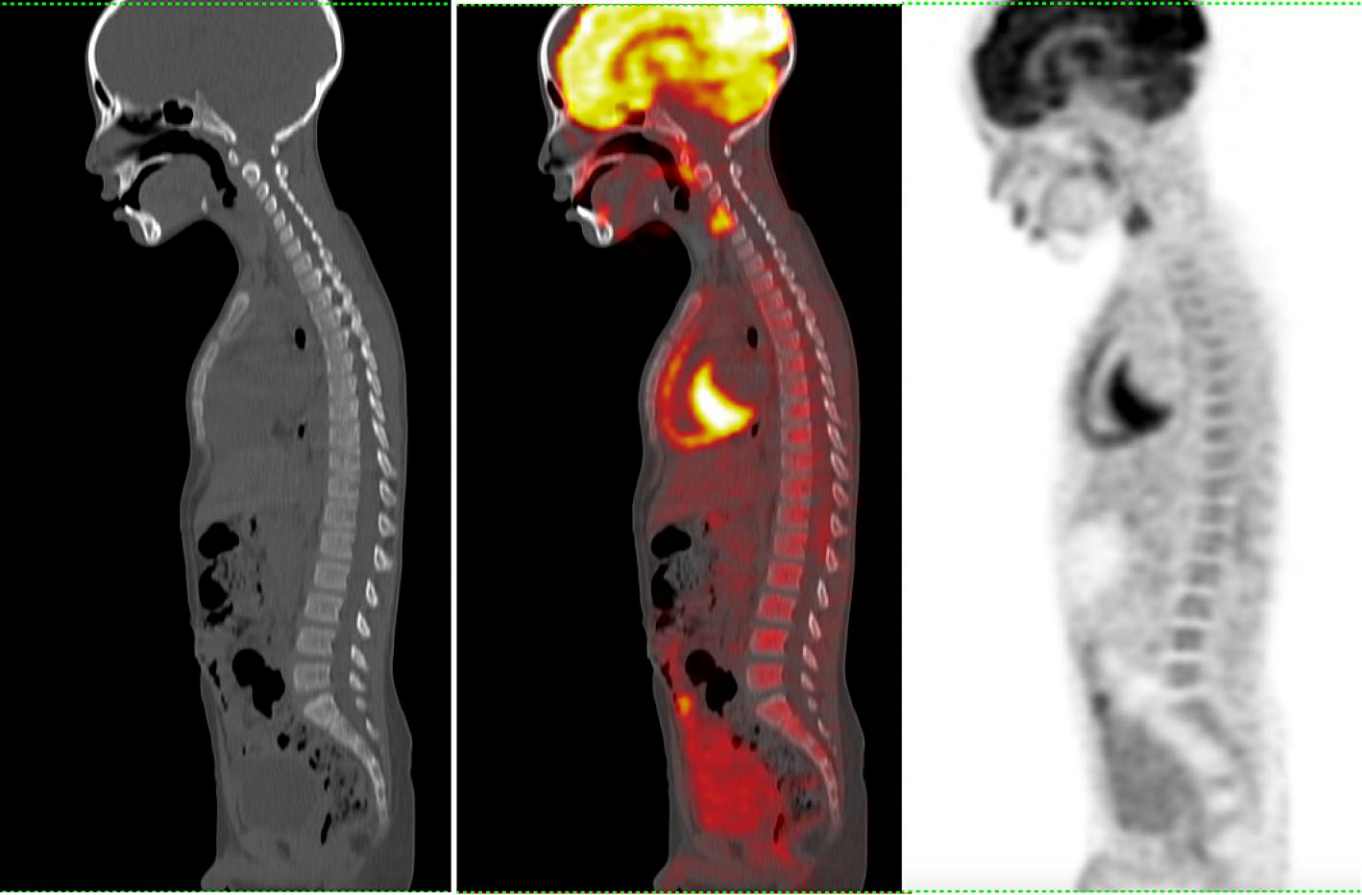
A 10-year-old male with Non-Hodgkin’s lymphoma (Burkett’s subtype) PET, fused PET/ CT images and CT images in sagittal view showing diffuse BM uptake. BMB was positive
